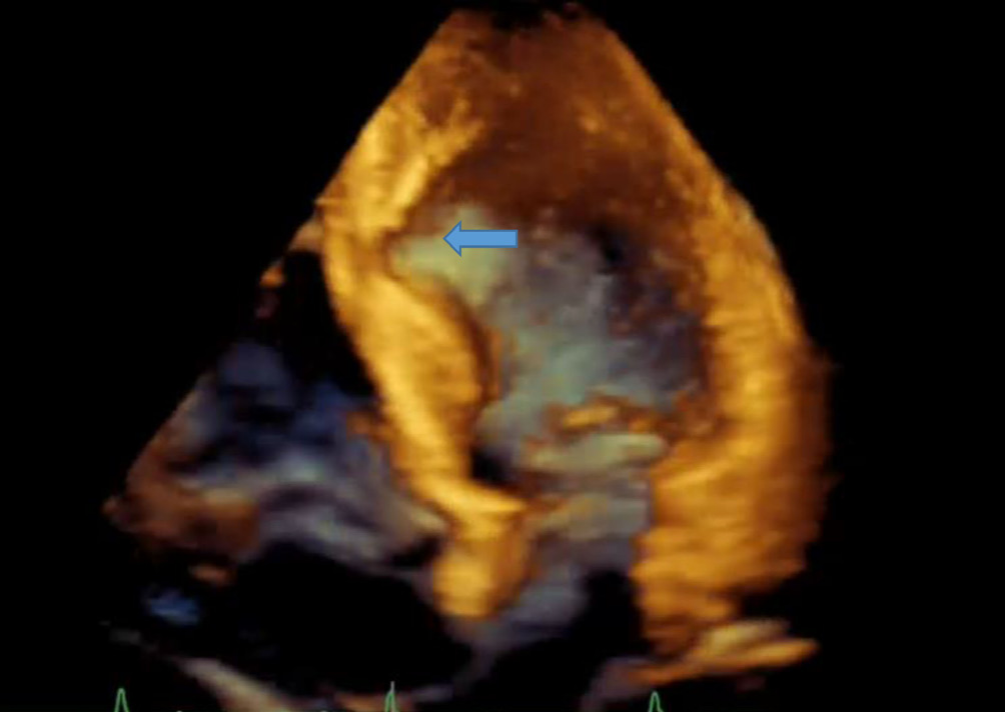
Four-chamber view of three-dimensional transthoracic echocardiography, showing a diverticulum in the mid inferoseptal wall (arrow)
